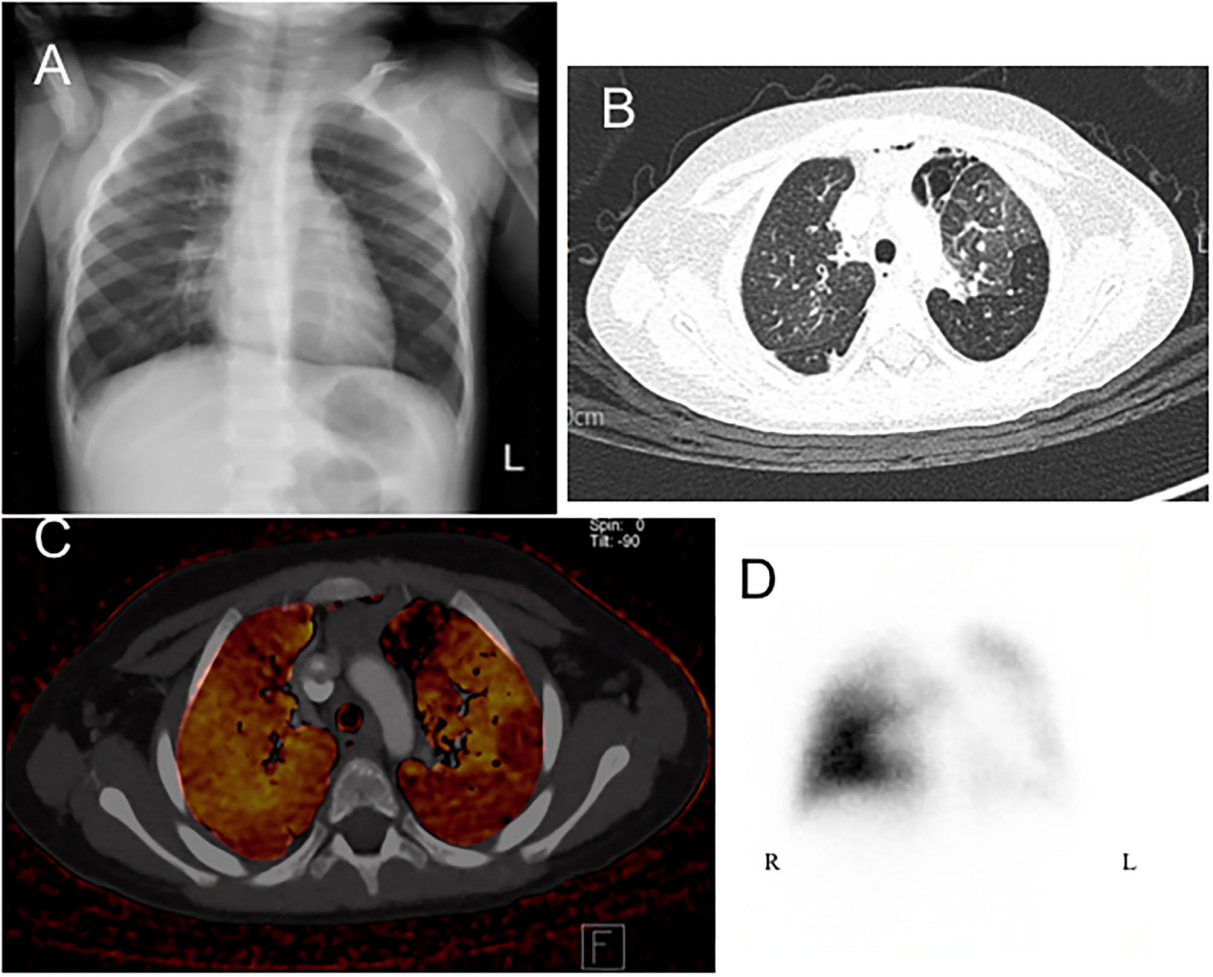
A 2-year-old boy presenting with tachypnea and dyspnea and receiving flexible bronchoscopy, which showed normal tracheobronchial appearance except much whitish sputum. (A) Chest X-ray revealed hyperinflation and attenuation of vascular marking in both fields. (B) Axial view of HRCT revealed mosaic pattern which are characterized by well-defined border, areas of decreased lung attenuation are associated with decreased pulmonary blood vasculature of DECT image (C). (D) Perfusion scintigraphy revealed marked lobar defects in right upper, left lower, and left upper lung fields. HRCT, high resolution computed tomography; DECT, dual energy computer tomography; R, right side; L, left side.
